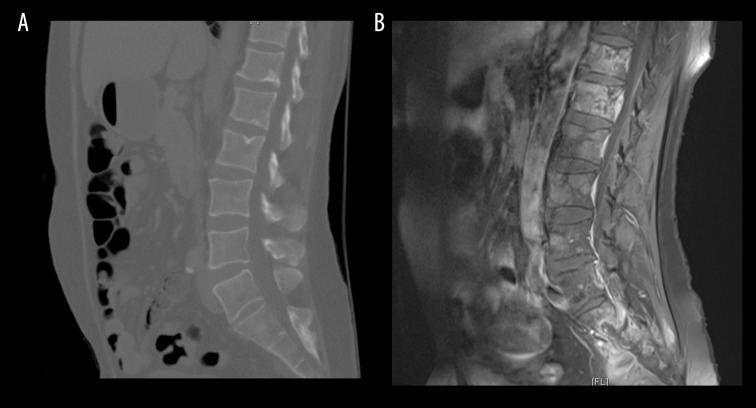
(A) CT lumbar spine image in sagittal section showing no evidence of metastatic disease. (B) T1, fat-saturated image in sagittal section showing extensive bony metastatic disease on MRI performed only one week after the CT of lumbar spine.
